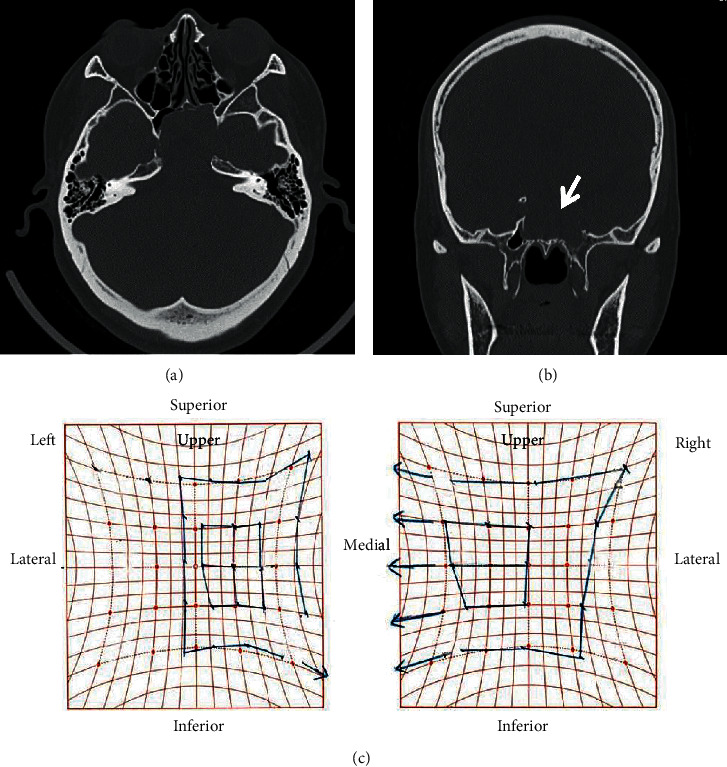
Noncontrast computed tomography imaging of the paranasal cavity and Hess screen test results at the emergency reexamination. CT images with (a) axial and (b) coronal views. The sphenoid sinus mucocele shows apparent growth, with increased impingement on the left superior orbital fissure (white arrow). (c) The Hess screen test confirms a disturbance in the left eye abduction and overaction in the right eye adduction.
